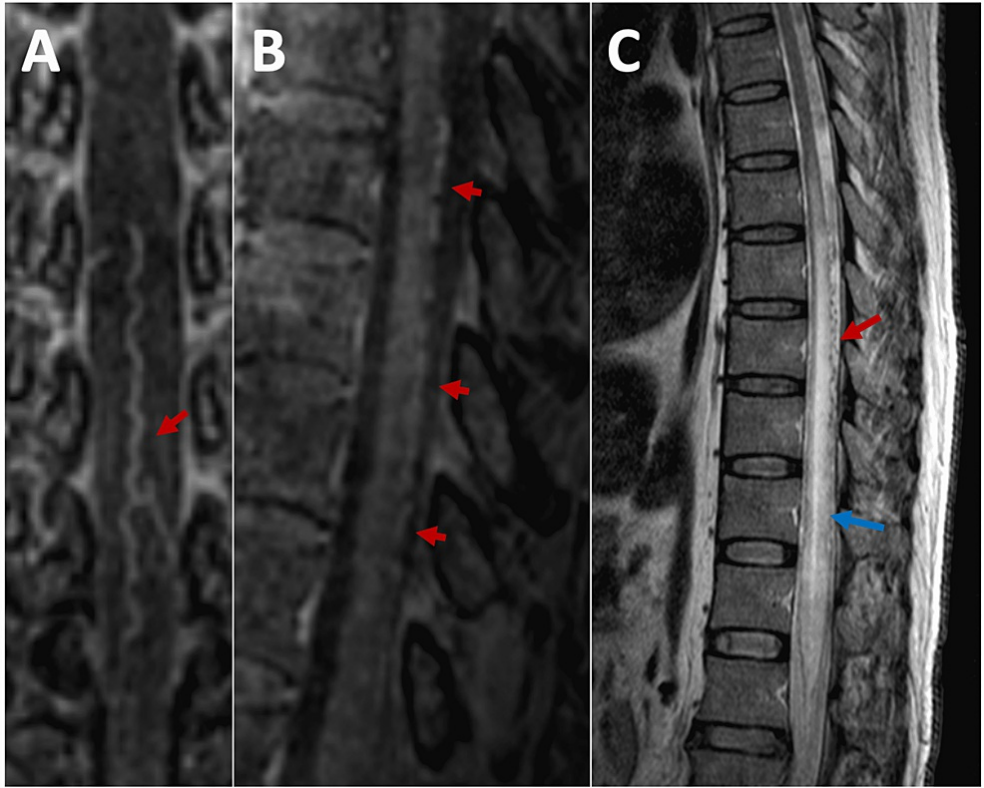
Spinal cord magnetic resonance images of cases 2 and 3. Case 2: (A) Coronal and (B) sagittal contrast images showing dilated and tortuous structures on the dorsal surface of the spinal cord. Case 3: (C) T2-weighted sagittal image showing dorsal myelopathy (blue arrow) and flow-void serpentine images on the dorsal surface of the spinal cord (red arrow).
